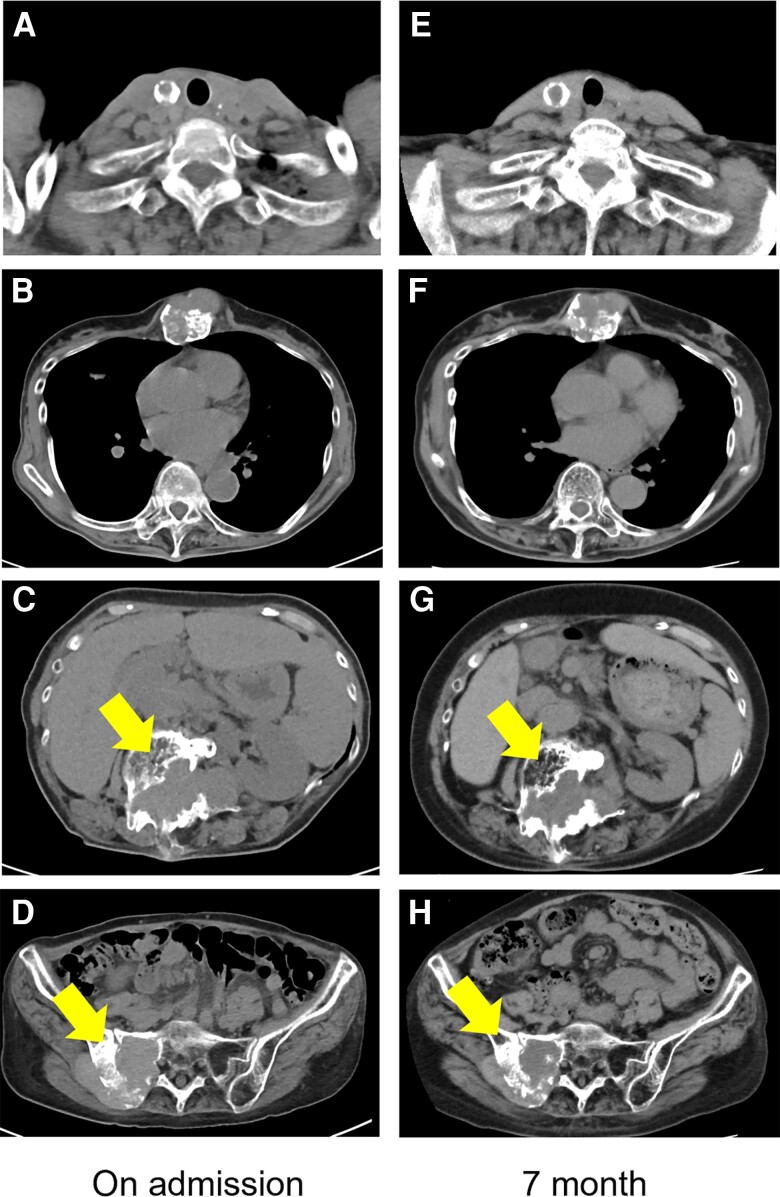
Comparison of computed tomography scans of the lesions in the A and E, thyroid; B and F, sternum; C and G, lumbar spine; and D and H, sacrum. There were no marked changes in the respective sizes of each lesion between A to D, admission and E to H, 7 months after admission.
